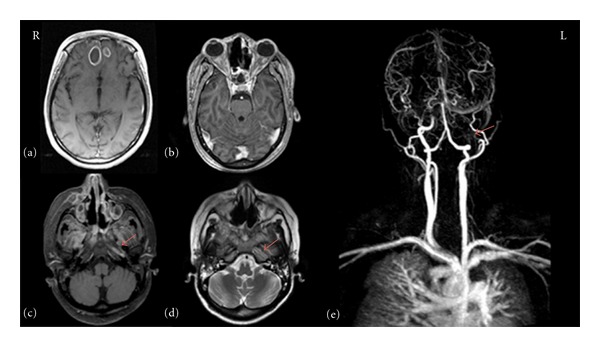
(a) MRI brain, axial T1 weighted image with gadolinium depicting anterior frontal bilateral abscesses. (b) MRI brain, axial T1 showing the orbital disease. (c) MRI brain, axial T1 demonstrating gadolinium enhancement around the left carotid artery canal. (d) T2 axial image demonstrating the absence of signal void at the left carotid artery. (e) MR angiography depicting complete occlusion of the left internal carotid artery cervical and cranial base segment.
